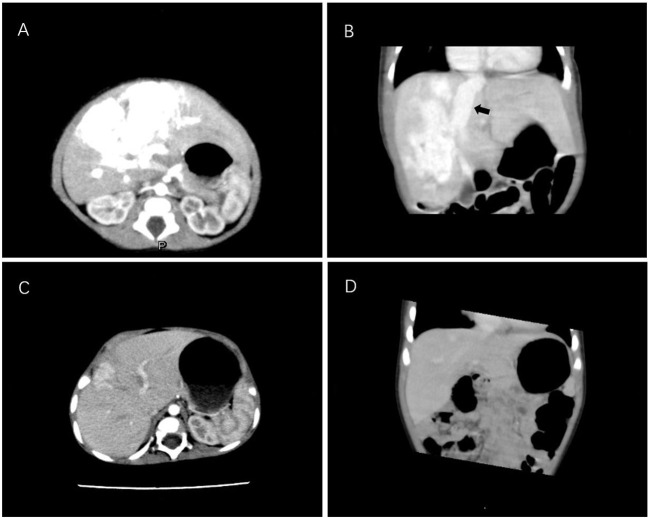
Enhanced CT scan of the liver in case 1. (A,B) CT images of the infant at 8 days old reveal a large arteriovenous fistula in the right hepatic lobe. The black arrow indicates the dilated hepatic vein. (C,D) A repeated CT scan after 9 months of propranolol treatment reveals that the hepatic arteriovenous fistula was significantly reduced. CT, computed tomography.
